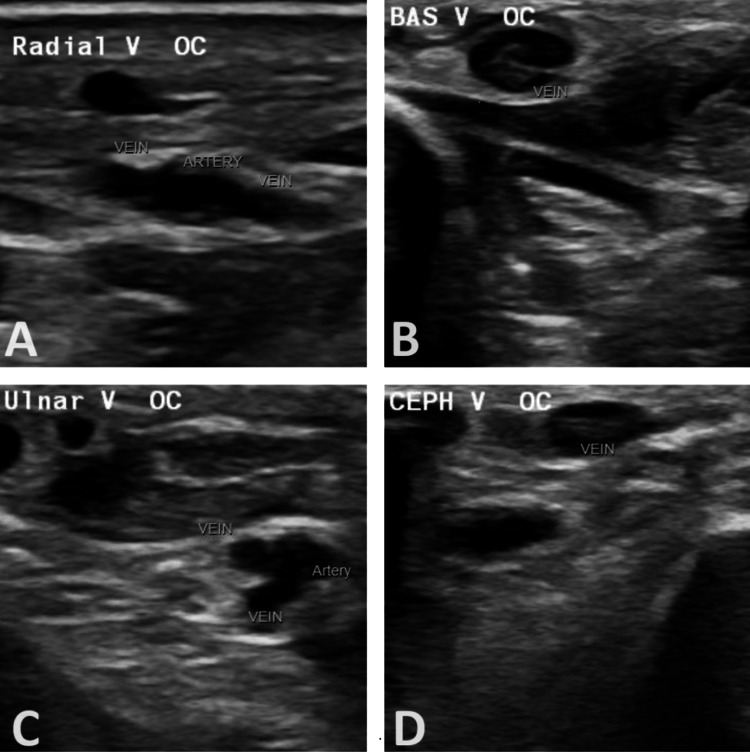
Venous doppler of the upper extremity. Occlusion of the distal upper arm and forearm radial [A], forearm basilic [B], forearm ulnar [C], mid-to-distal upper arm and forearm cephalic veins [D] by echolucent thrombi.
